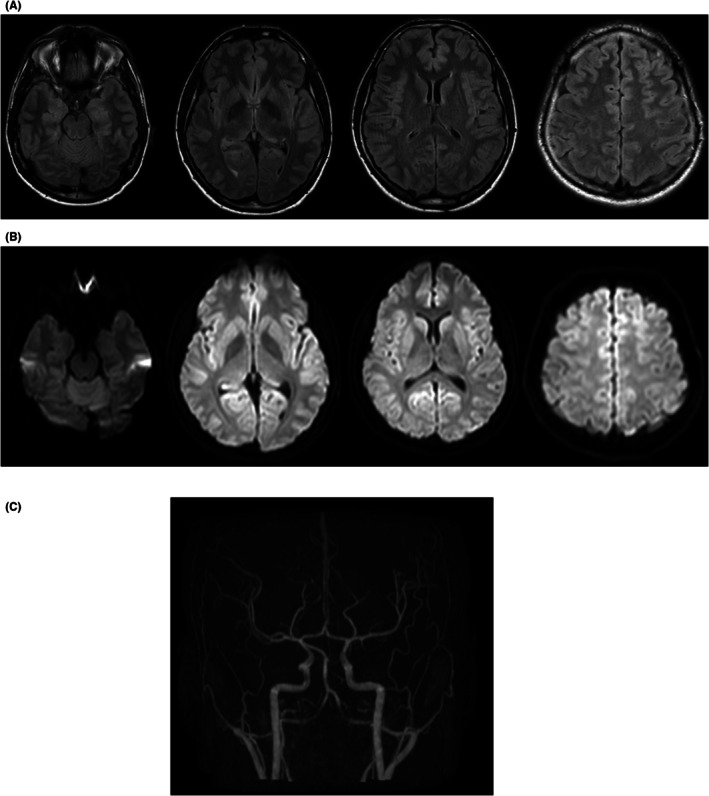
MRI without contrast was performed following the admission to the emergency room to rule out a stroke. We present progressive slices from ventral to dorsal from fluid attenuated inversion recovery (FLAIR) (A) and diffusion weighted imaging (DWI) B1000 (B) sequences, as well as Time‐of‐Flight (ToF) angiography of intracranial vessels (C), without pathological findings.
